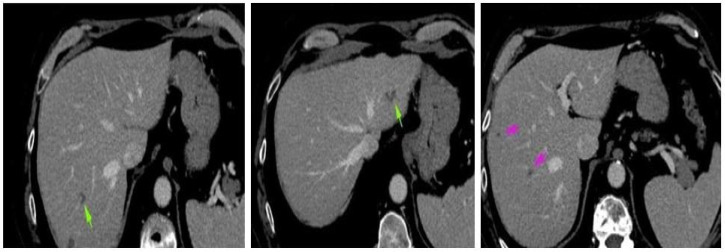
Abdominal CT scan: hepatic metastases.
